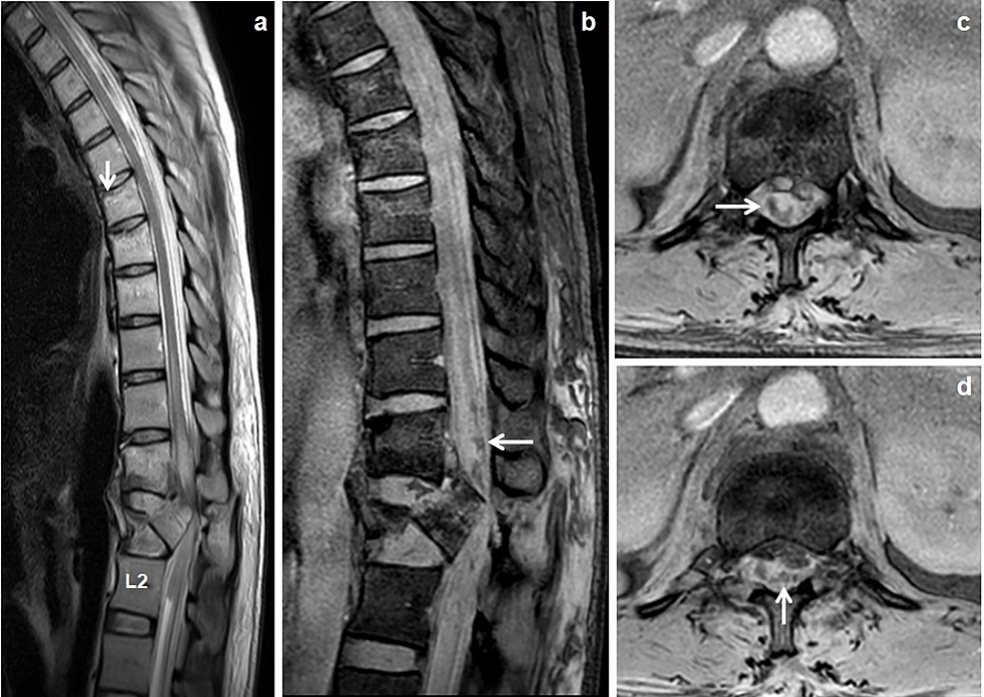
Vertebral burst fractures. Day 1 MRI scan of 41-year-old female patient with history of RTA. Sagittal T2W image (a) showed displaced burst fractures of L1 vertebra retropulsed into spinal canal and teared the longitudinal ligaments, crushed injury to the adjacent intervertebral discs and partial cord transection with long segmental cord edema up to D10 vertebral level. Occult fractures/bone contusions observed in D7, D8 and D9 vertebrae (↓arrow). Sagittal mFFE image (b) showed extensive cord hemorrhages in the lower dorsal cord (←arrow). Axial mFFE images (c & d) showed hemorrhages within the central (↑arrow) and para-central (→) locations of the cord. mFFE: multiecho fast field echo; RTA: road traffic accident.
